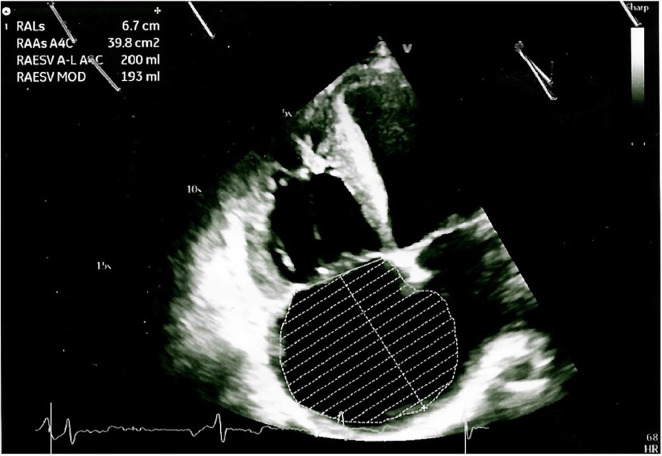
Doppler echocardiography showing cardiac signs of PAH, including right ventricular dilatation, severe tricuspid regurgitation, and a severe right atrial dilatation.
